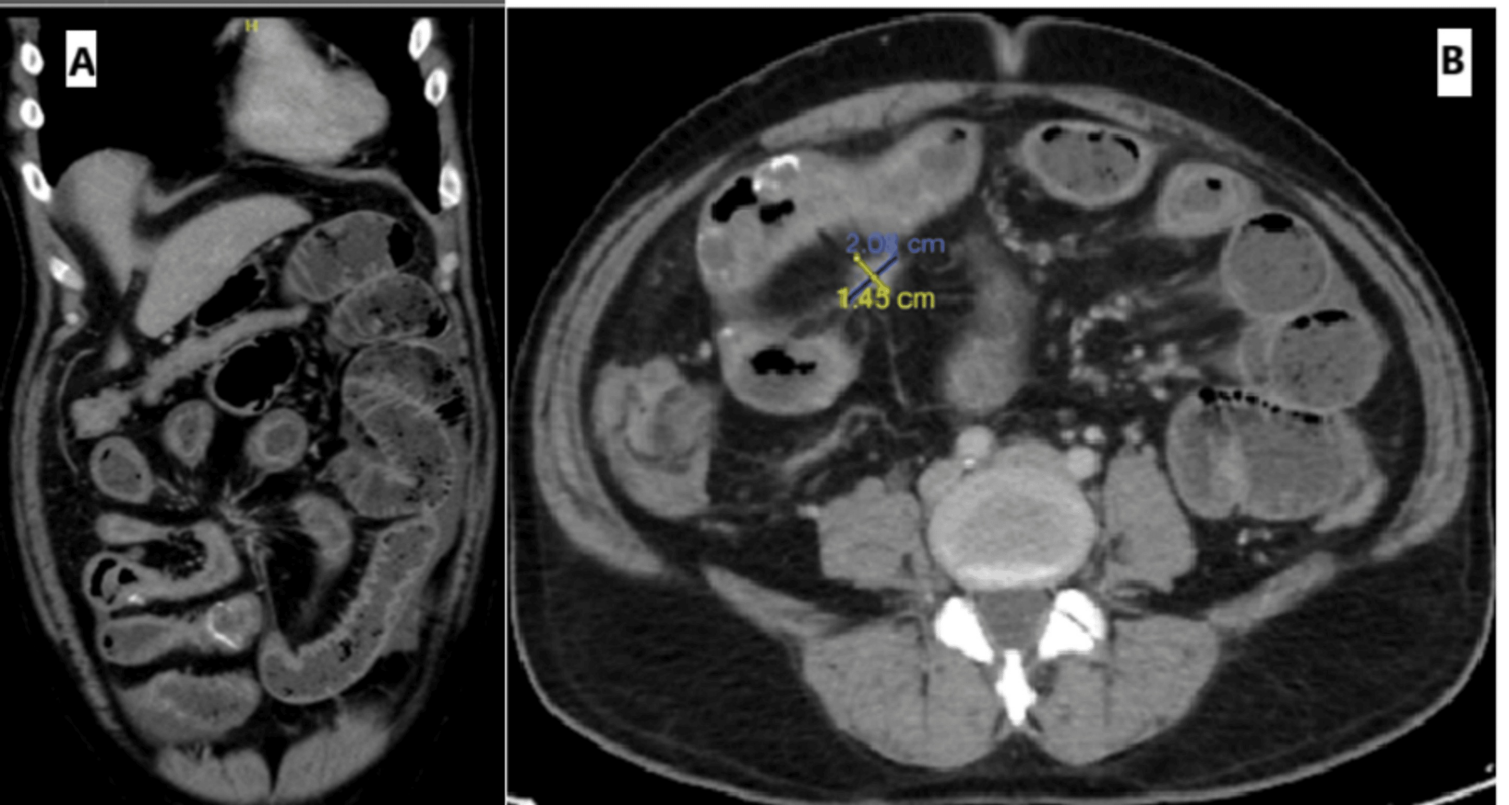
(A) Coronal CT image showing eggshell calcification at the distal ileal loops and (B) axial CT image showing a cauliflower-shaped mass at the root of the mesentery
